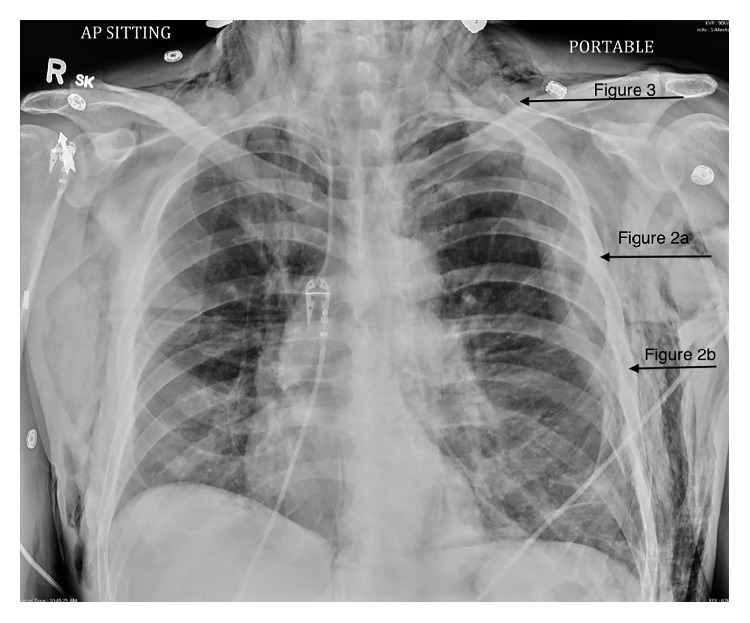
Anteroposterior chest radiograph demonstrating subcutaneous emphysema. Anatomic locations of Figures 2(a), 2(b), and 3 are indicated.
